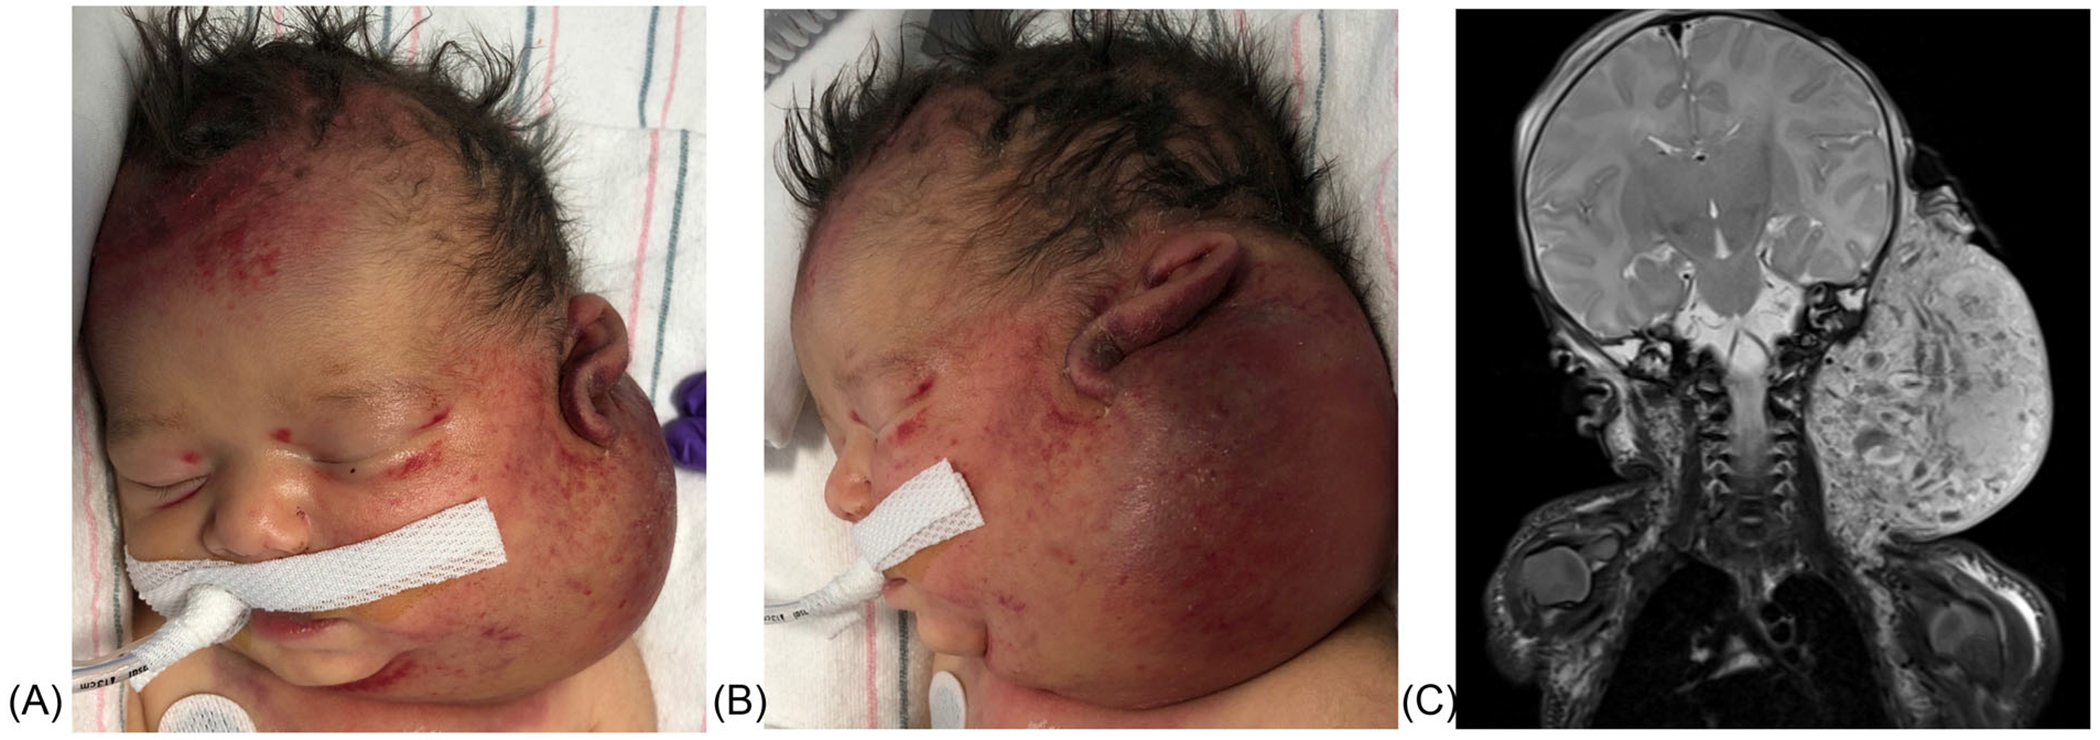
Kaposiform hemangioendothelioma (KHE). (A) There is a large, firm, and indurated mass extending over this infant’s left ear, neck, and cheek. Note the petechiae and purpuric discoloration overlying the skin in this patient with thrombocytopenia and hypofibrinogenemia. (B) The lesion was present at birth and involves the skin, parotid gland, sternocleidomastoid muscle, and the deep spaces of the neck. This patient was intubated due to concern for mass effect of the lesion compressing the patient’s airway. (C) Coronal T2 weighted magnetic resonance imaging (MRI) with fat saturation demonstrates a highly infiltrative mass extending from the left scalp and periauricular region inferiorly into the upper anterior chest wall. The mass crosses fascial planes and extends along the paravertebral muscles and retropharyngeal space. There is also involvement of the nonarticular portion of the left temporomandibular joint, which appears deformed and possibly subluxed
